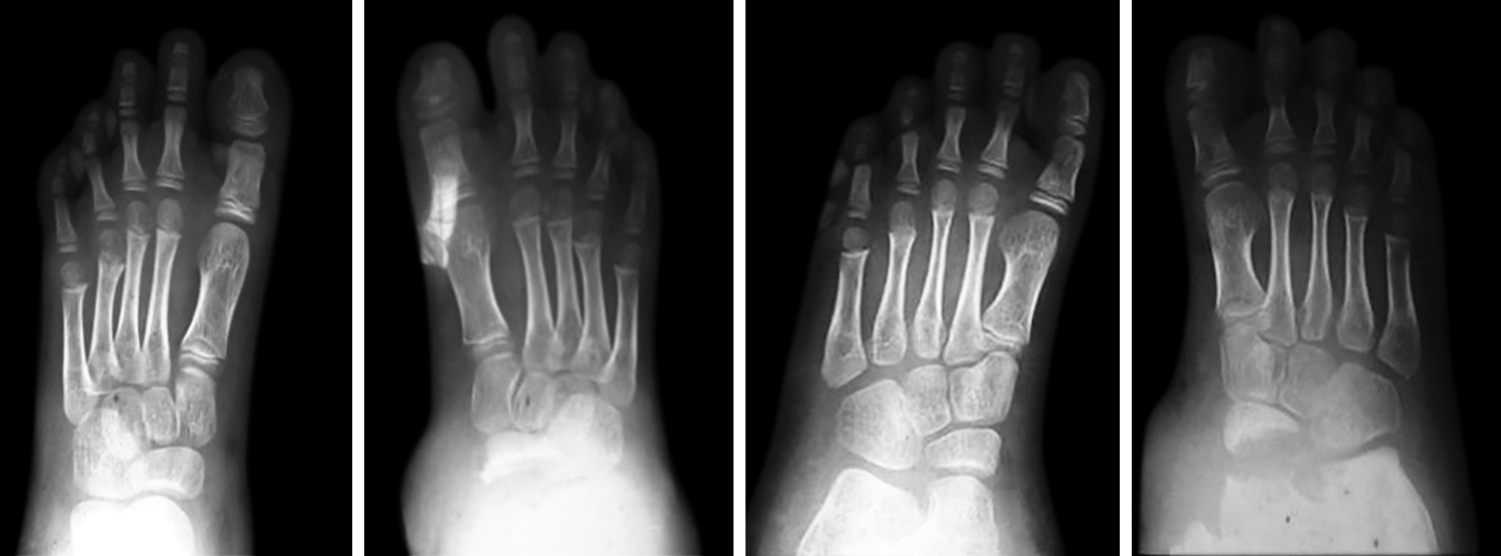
Radiographs of the feet revealed Charcot’s joint
